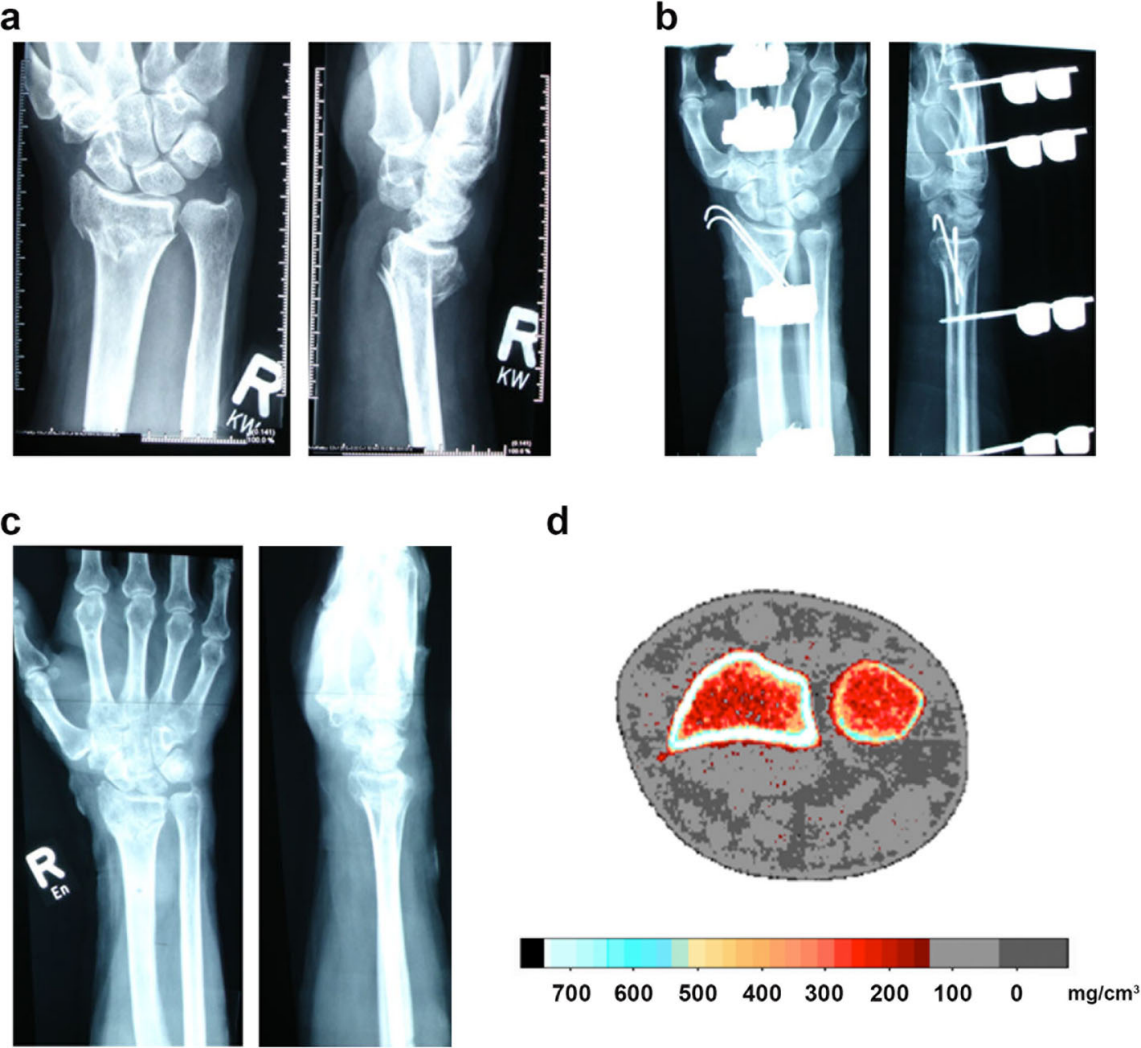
a: Preoperative X-ray photograph in anterior-posterior and lateral view of the right arm of the patient. b: Stabilisation with external fixateur and additional k-wires after 2 weeks postoperatively. c: Postoperative X-ray photograph in anterior-posterior and lateral view of the right arm of the patient after 6 weeks. d: Example of a pQCT analysis of a cross-section of the forearm at measurement height. Color scale below the cross section indicates the range of the calculated bone density
